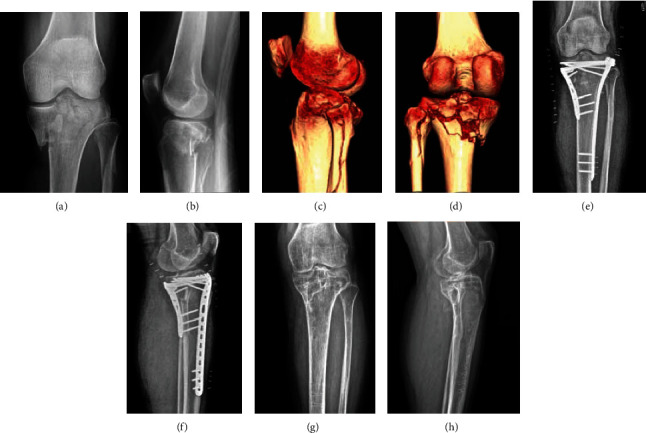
A tibial plateau fracture case with type 3 fibular column injury: (a, b) preoperative X-ray; (c, d) preoperative CT; (e, f) postoperative X-ray; (g, h) X-rays after removal of implant.
